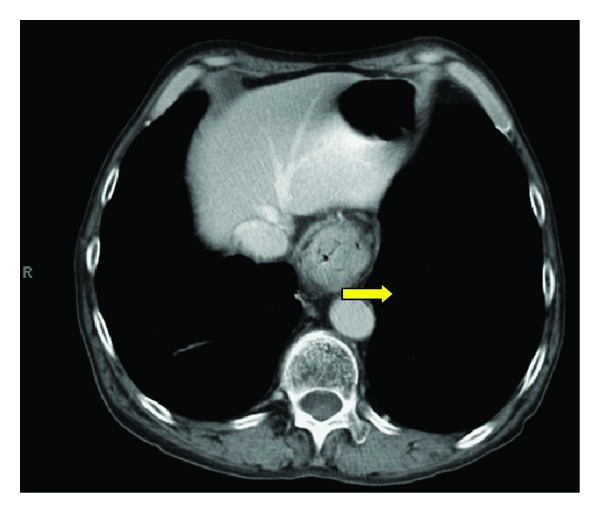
CT of chest showing a near-obstructing mass in the distal esophagus (yellow arrow).
